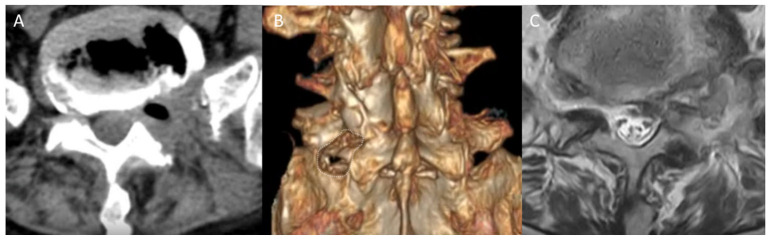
Postoperative imaging evaluation. (A) Axial CT scan showing the extent of the bony decompression. (B) Three-dimensional CT reconstruction; the white dotted line delineates the area where intraoperative bone resection (bony work) was performed while preserving the facet joint. (C) Postoperative axial T2-weighted MRI demonstrating successful removal of the offending herniated disc and lumbosacral ligament, along with partial resection of the pseudoarticulation, resulting in significant expansion of the L5 nerve root space.
